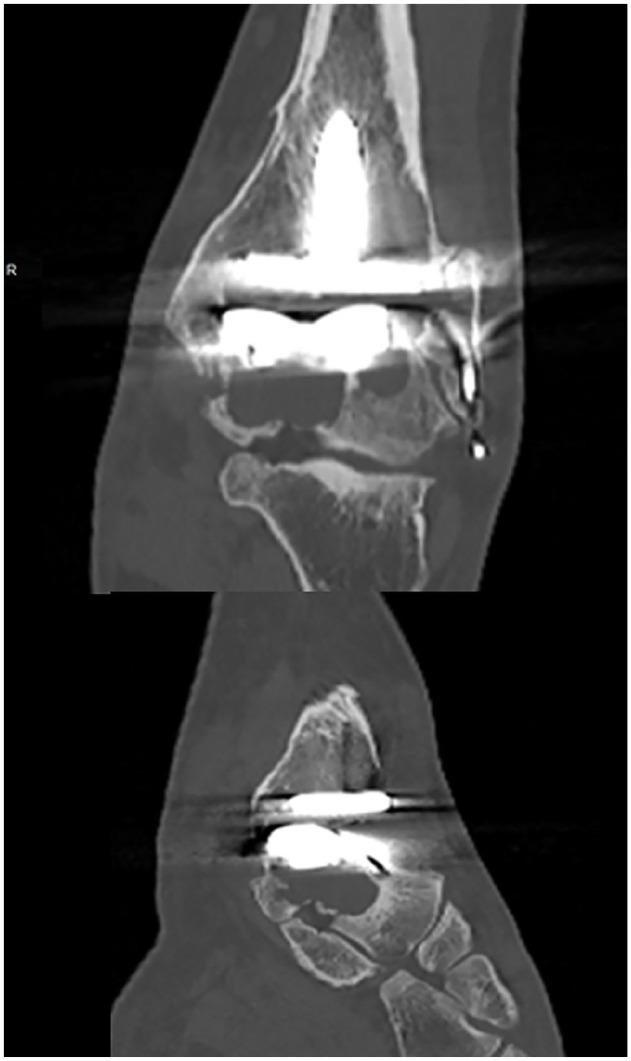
Preoperative coronal and sagittal computed tomographic slices of the case in Figure 7. In this case there were large talar cysts throughout the height of the talus and involving the anterior plafond.
